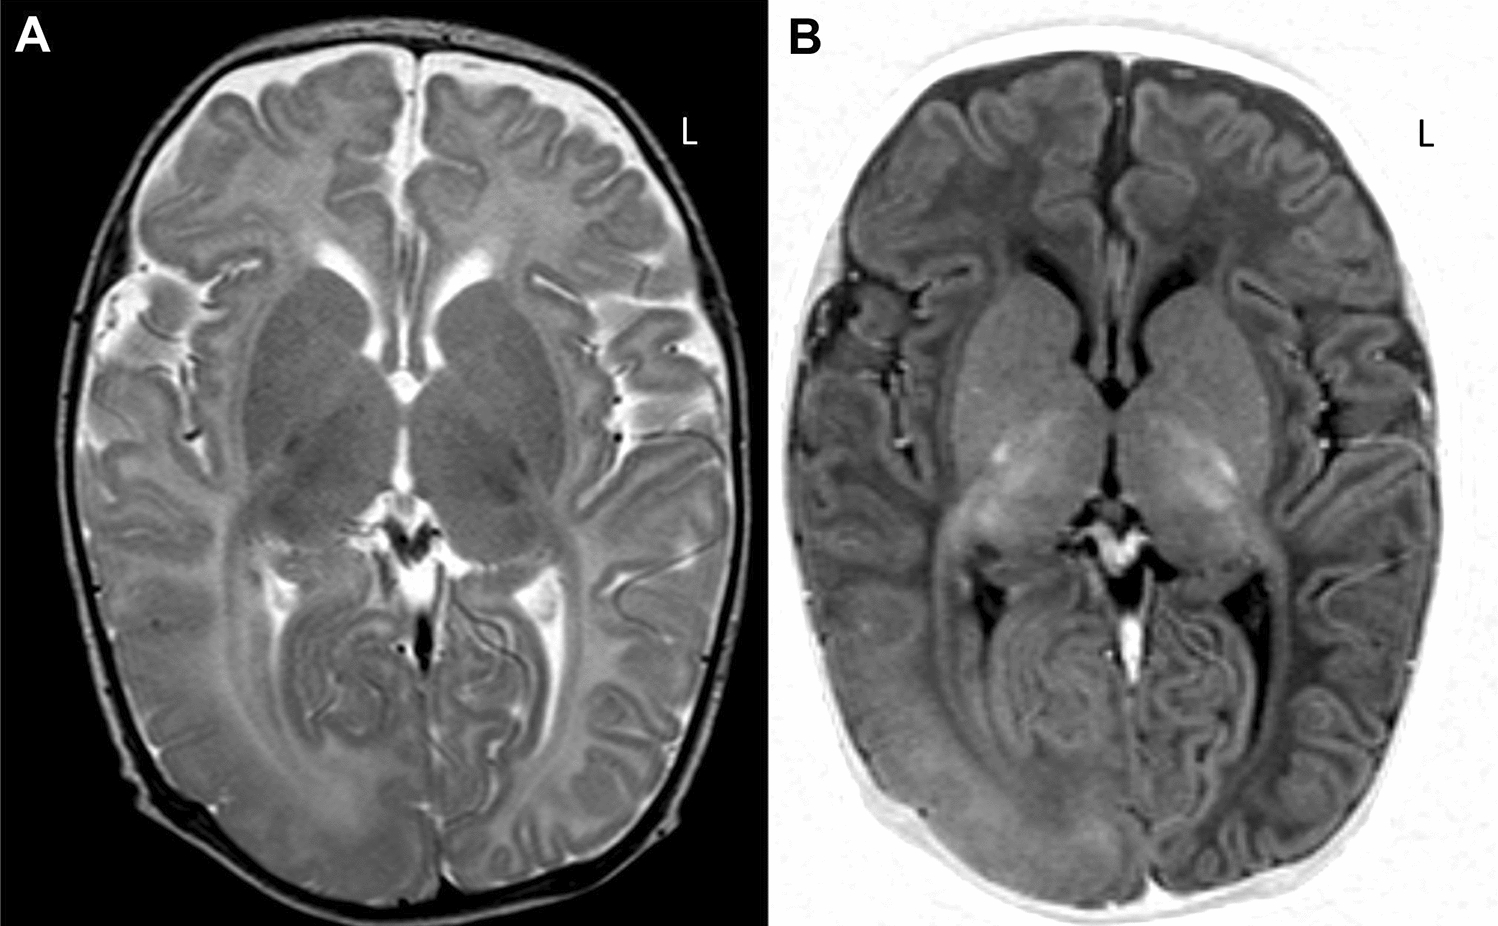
Hemimegalencephaly MRI. MRI from a female at age 2 months. A, B T2w and T2w images showing enlargement of the right occipital and adjacent posterior temporal/parietal lobes with diffuse thickening of the cortex, abnormal shallow overlying sulcation and underlying white matter signal abnormality. Findings are consistent with posterior quadrantic dysplasia (localised or hemi hemimegalencephaly)
